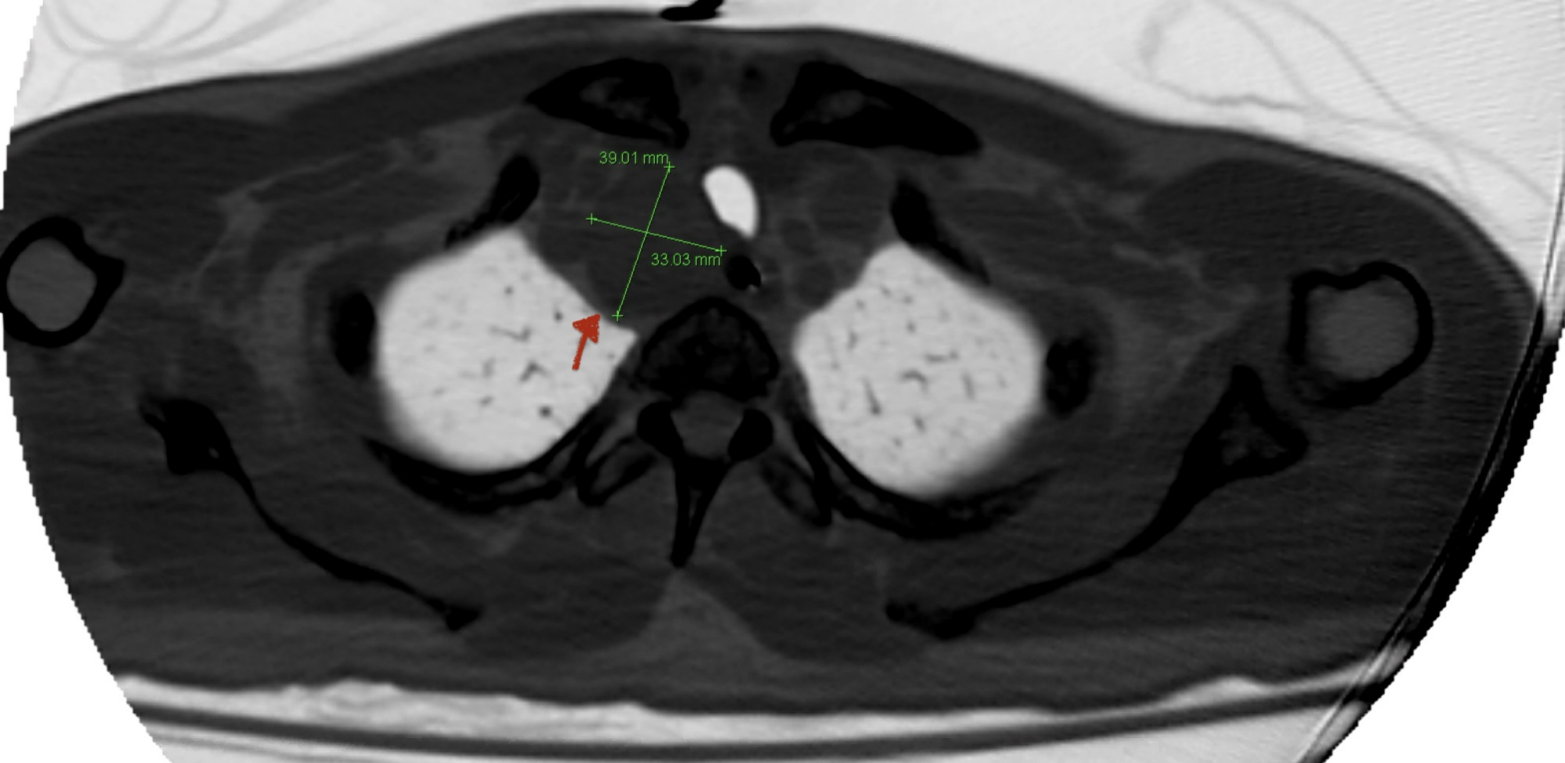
Chest CT scan A 39x33 mm mass located at the right retroclavicular location that indented and deviated the trachea to the left side.
